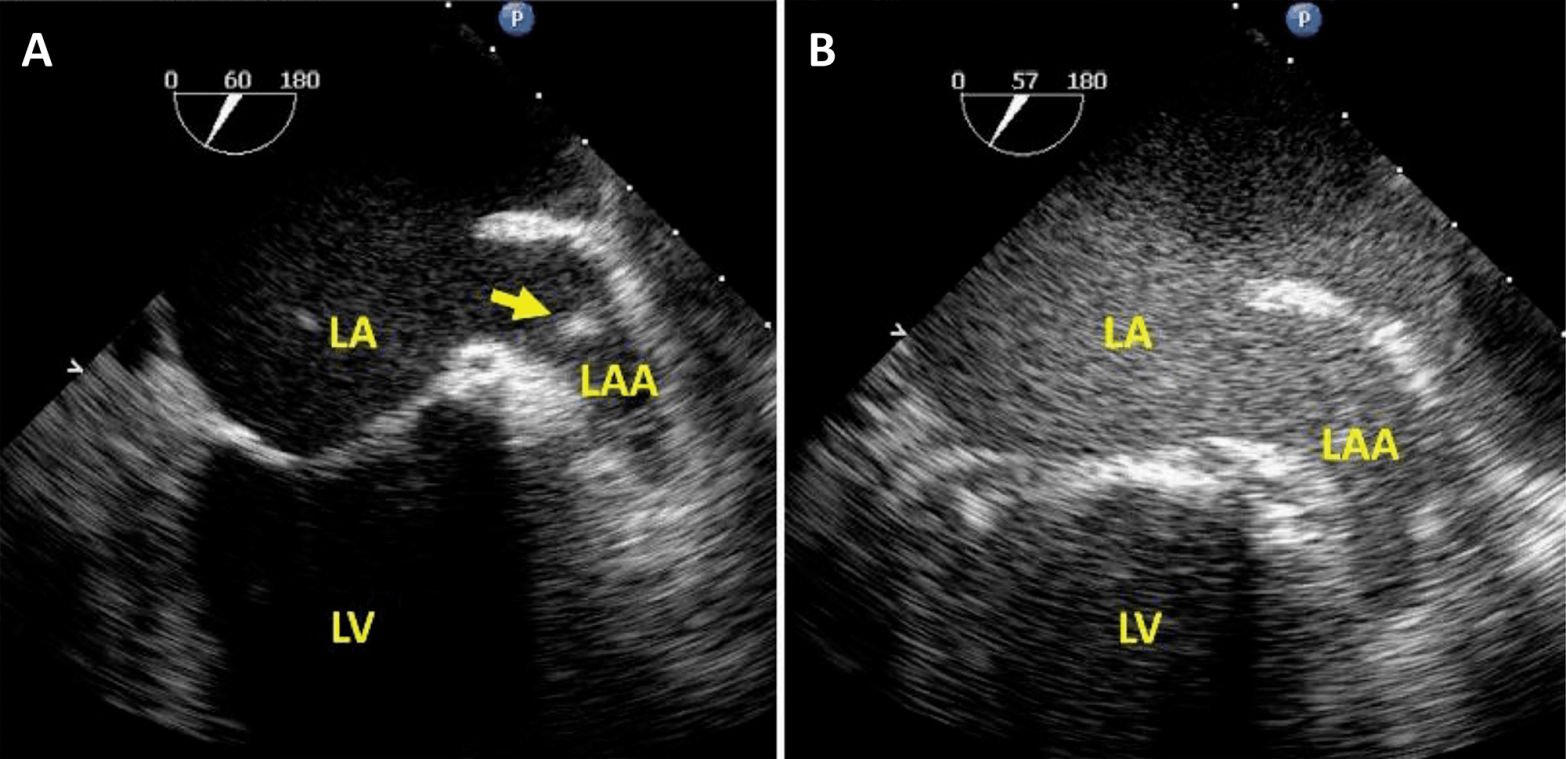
Ultrasound-enhanced TEE used to facilitate the safety of an
